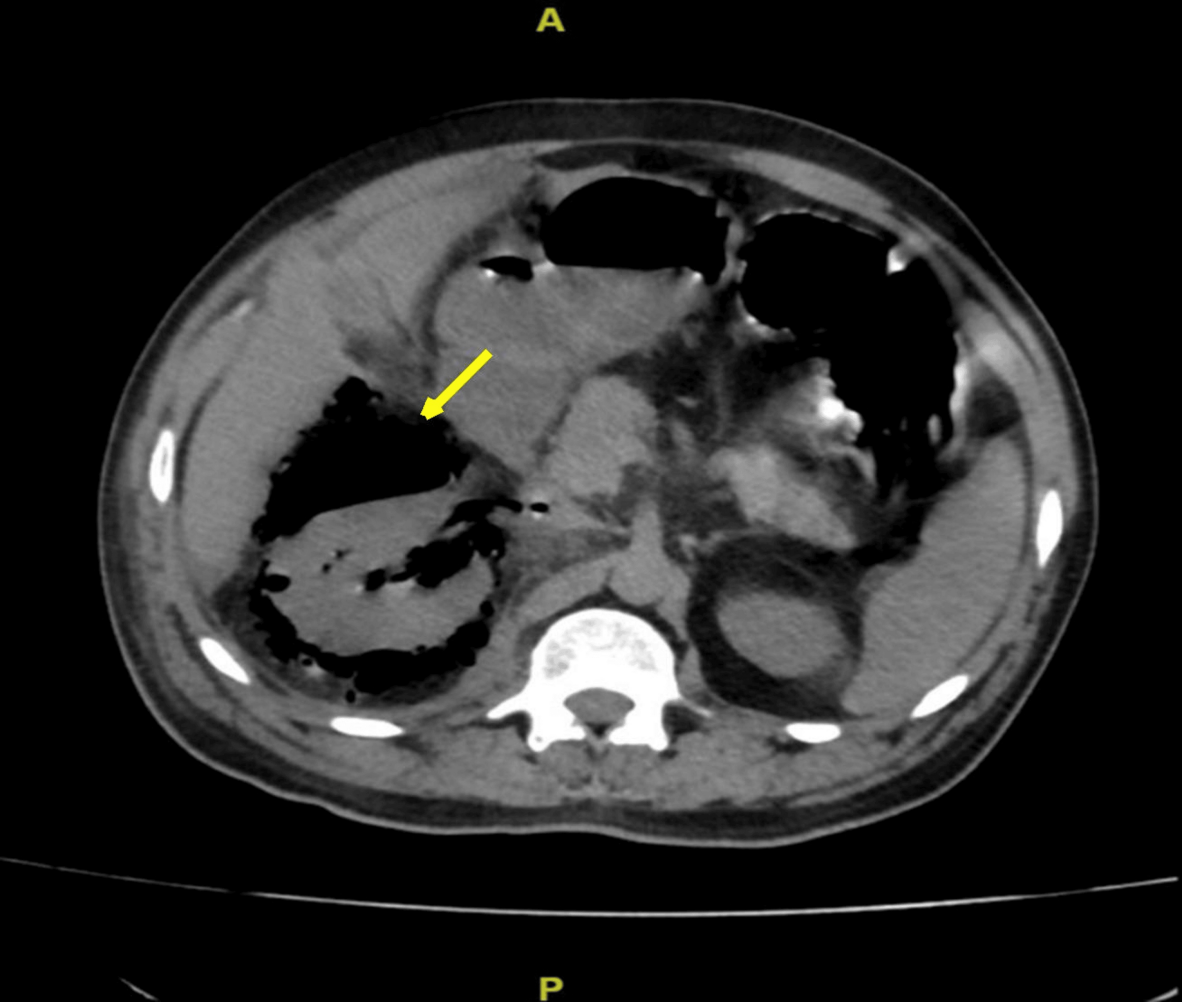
Computed tomography image (axial section) of the abdomen showing extensive gas within the right renal parenchyma and perinephric space and extending into the pararenal space, consistent with emphysematous pyelonephritis grade 3B
